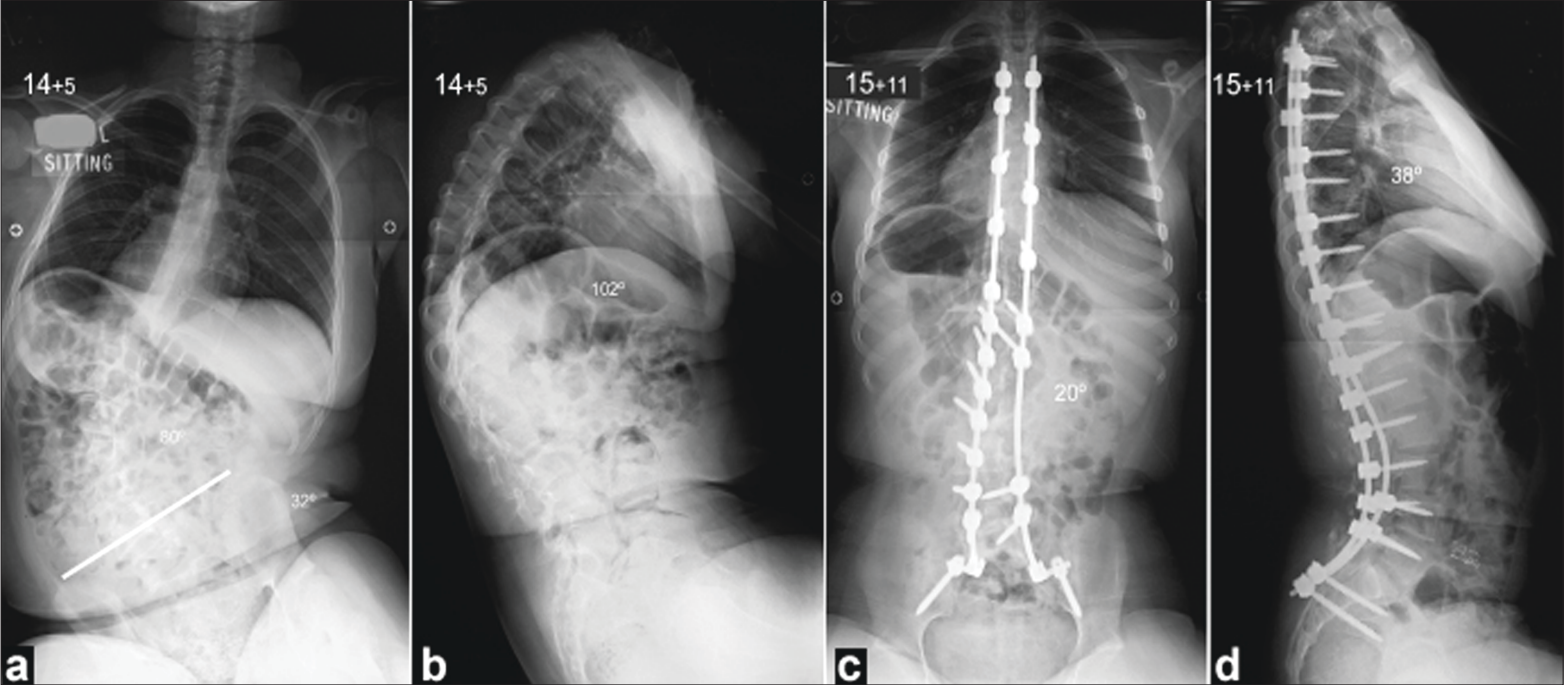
Preoperative posteroanterior (a) and lateral (b) radiographs of the spine show a severe kyphoscoliosis with associated pelvic obliquity on the right side, as well as rib impingement. The patient underwent a posterior spinal fusion with the use of pedicle screw instrumentation (c, d), which resulted at an excellent correction of the deformity as well as a balanced spine with level pelvis
